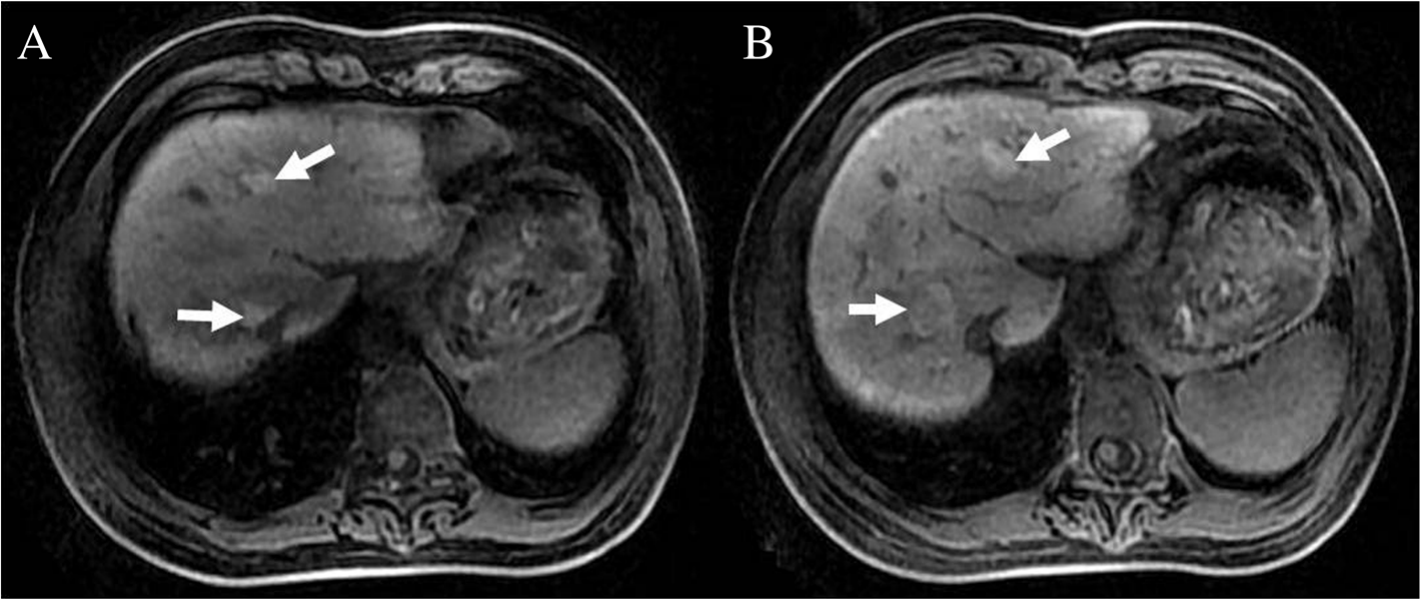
Delayed hepatobiliary-phase of magnetic resonance imaging (MRI). Following administration of gadobenate dimeglumine, additional hepatic tumors were found (A and B, white arrows).
